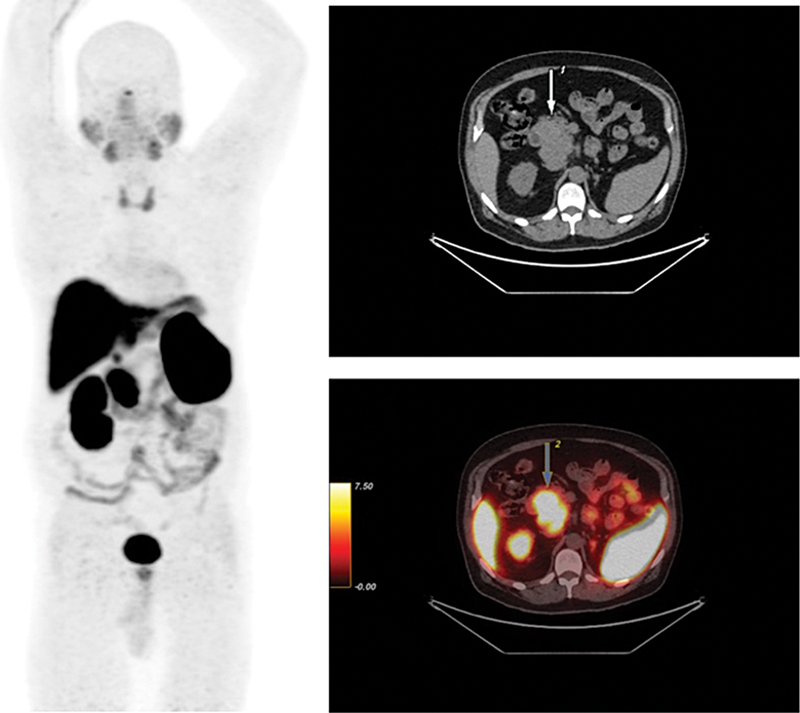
Maximum intensity projection, transaxial CT and fused PET/CT images demonstrated pathologically intense
